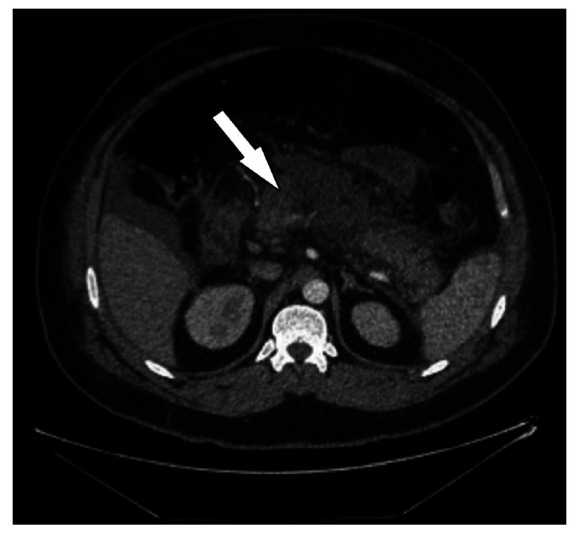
Computed tomography scan of a patient with peripancreatic fluid collection (arrow).
